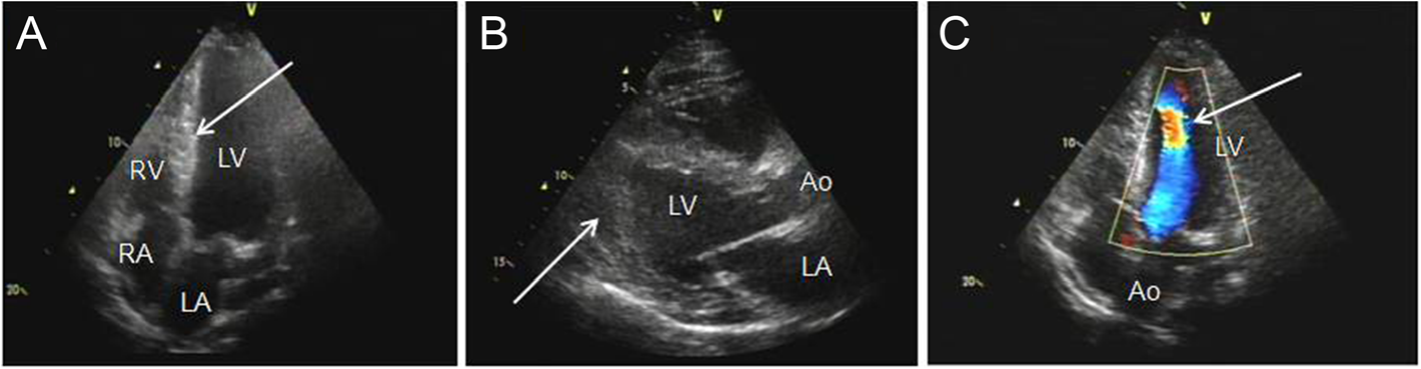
Two-dimensional echocardiograms. (A) Apical four-chamber view of the left ventricle (LV) in telediastole with mid-apical hypertrophy (arrow). (B) Longitudinal, long axis view of the LV shows hypertrophy confined to the mid-apical and apical segments of the ventricular septum (arrow). (C) Apical five-chamber of the LV shows the intraventricular gradient (arrow). Ao, Aorta; LA, Left atrium; RA, Right atrium; RV, Right ventricle.
